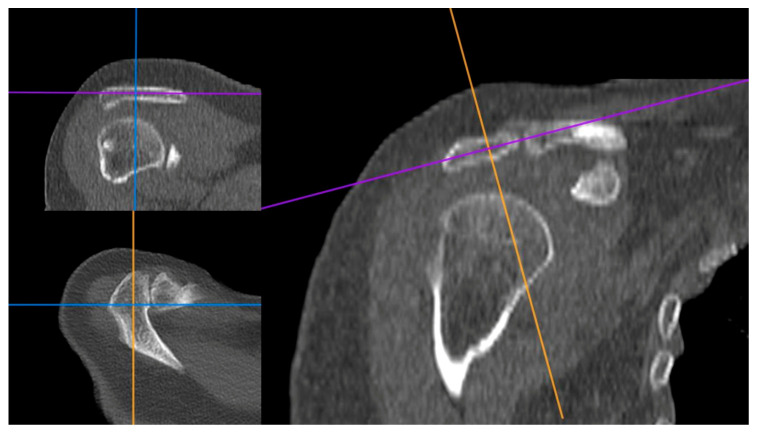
Method for finding the points where the acromion HU value measurement is taken. The center of the acromion bone in axial cut was determined using a coordination facilitated through reformatted coronal and sagittal cut in CT images. Purple line: Axial plane; Blue line: Coronal plane; Orange line: Sagittal plane.
